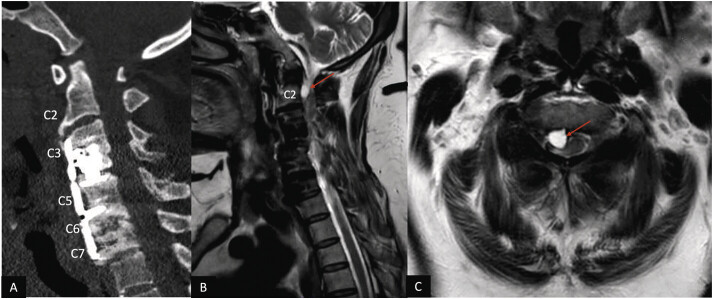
Preoperative sagittal CT scan (
